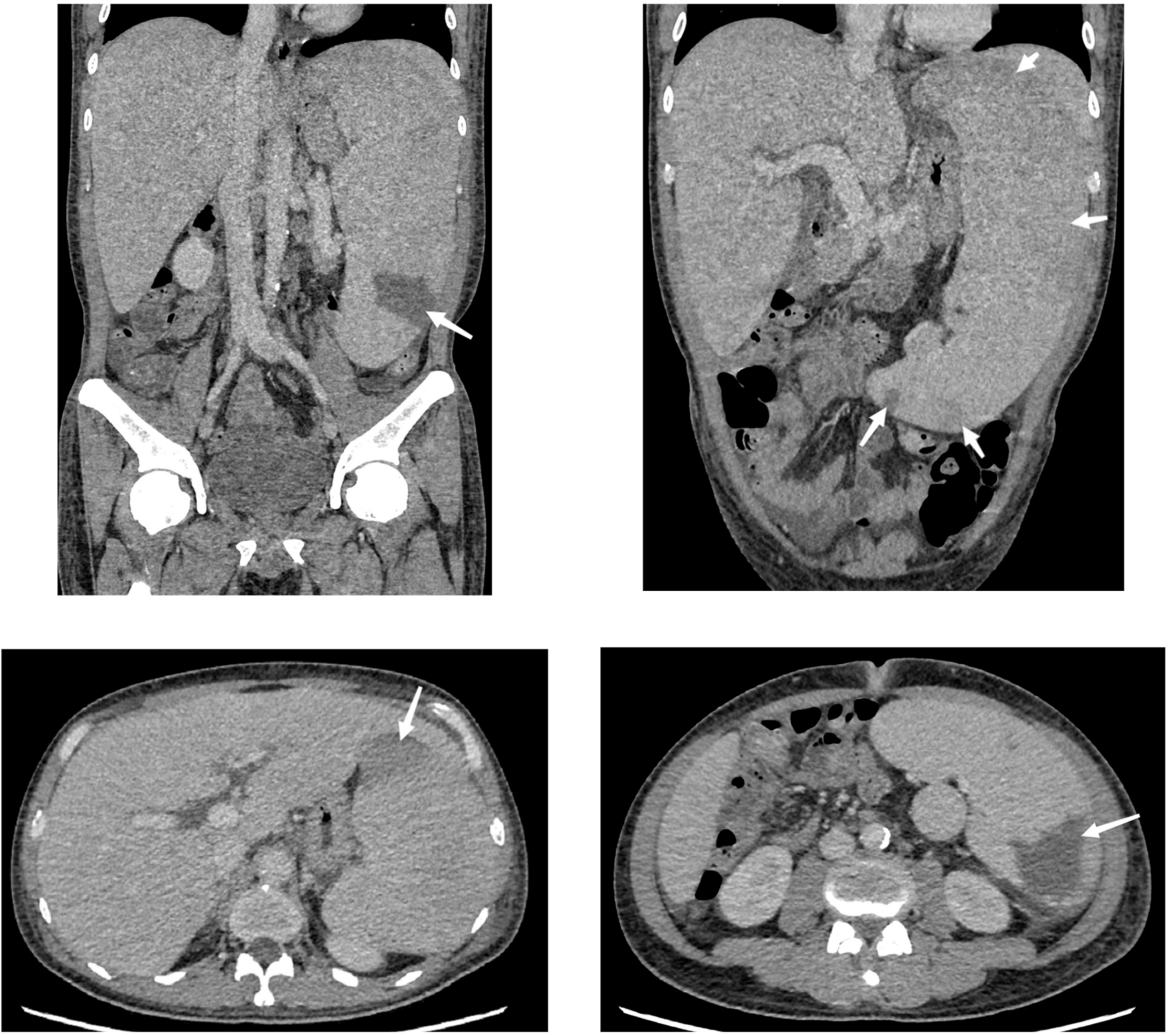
Abdominal contrast-enhanced computed tomography cross-section. The liver is fully voluminous and has a uniform density. The spleen is significantly enlarged and thickened, with the thickest part measuring approximately 82 mm. The edges are rounded, and the lower border of the spleen is below the lower border of the liver. There are multiple hypointense or non-enhancing wedge-shaped areas near the edge of the spleen, suggesting splenic infarction (arrows). A nodular accessory spleen is also observed along the inner edge of the spleen.
